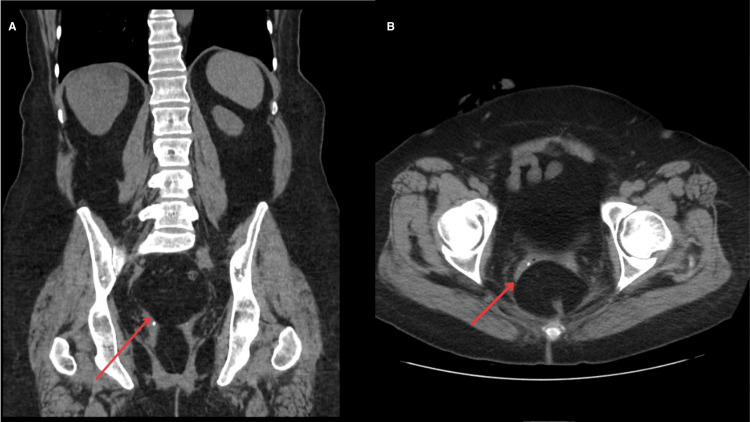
CT scans WOC pre-tumor Coronal (A) and axial (B) CT scans WOC taken in 2010 showing no gynecological involvement (red arrows). CT: computed tomography; WOC: without contrast
